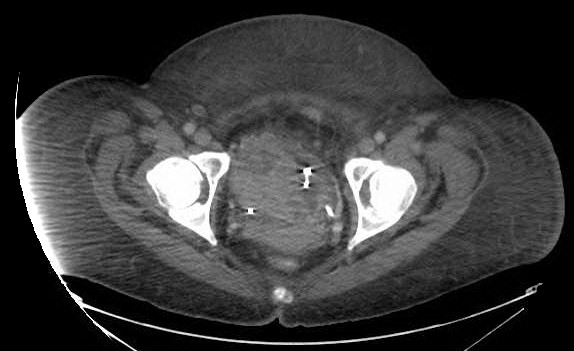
Computed tomography scan of the pelvis showing large nodular bladder mass with heterogeneous enhancement, prior to cystectomy. Exchange are most extensive along the right bladder wall though is also seen along the left bladder wall and fundus.
